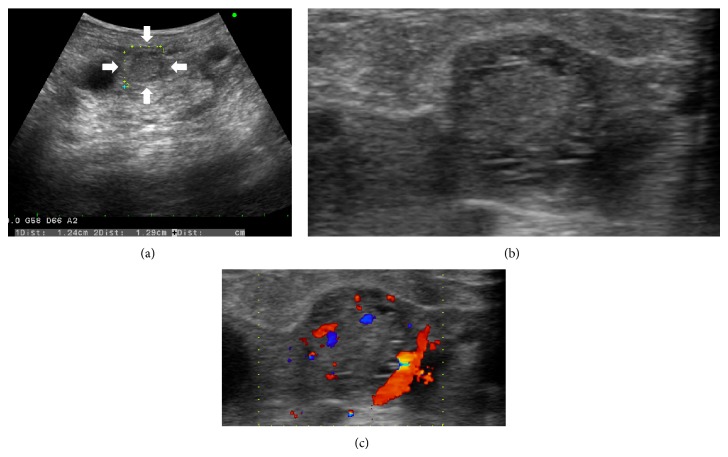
Ultrasonography images. (a) and (b) Ultrasonography revealing a solid tumor with a hypoechoic rim in the superficial cortex of the kidney graft (*white arrows*). (c) The hypervascular nature of the kidney graft tumor is confirmed on a Doppler image.
